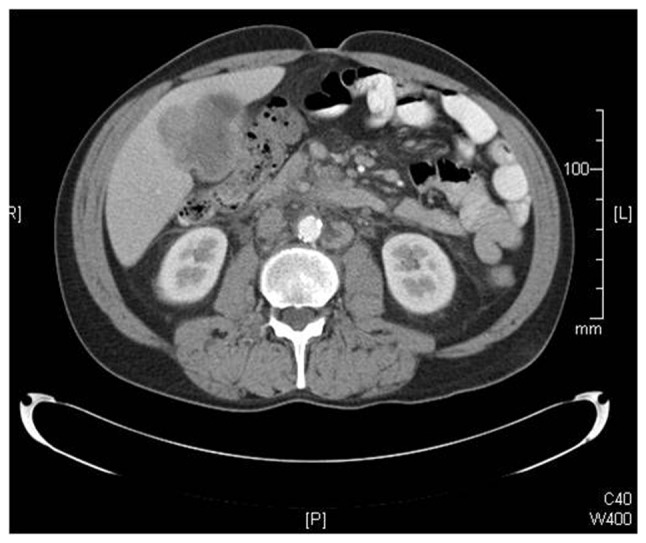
Abdominal CT scan showed a heterogeneous gallbladder fossa mass invading the right hepatic lobe and enlarged periportal, pericaval, and periaortic lymph nodes.
